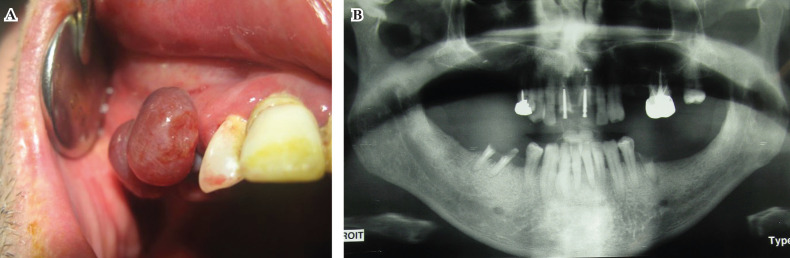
Clinical view of the violaceous, exophytic mass of the right maxilla oral mucosa mass (A), Panoramic radiograph for evaluation of the presence of infectious dental apical lesions (B).
